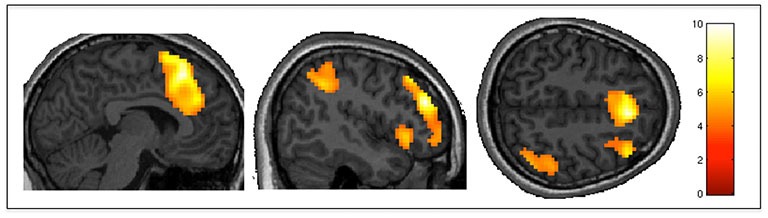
Action selection vs. command following. AS (participants selected their movements), as compared to CF (movements were determined by experimenters) elicited greater activity in the middle frontal gyrus, pre-SMA, somatosensory association cortex and insula. Results are thresholded at FWE-corrected *p* < 0.05 for cluster level activation, and overlaid on an anatomical T1-weighted image.
